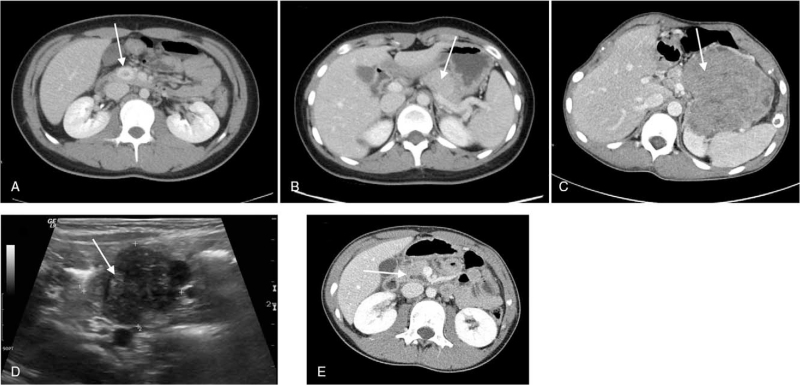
Preoperative imaging (each tumor is annotated with a white arrow). A: Patient 1, 1.9 × 1.4 cm; B: Patient 2, 3.3 × 2.9 cm; C: Patient 3, 10.0 × 9.8 cm; D: Patient 4, 2.3 × 1.9 cm; and E: Patient 5, 1.3 × 0.9 cm; Due to the small age of patient 4, computed tomography was not performed.
